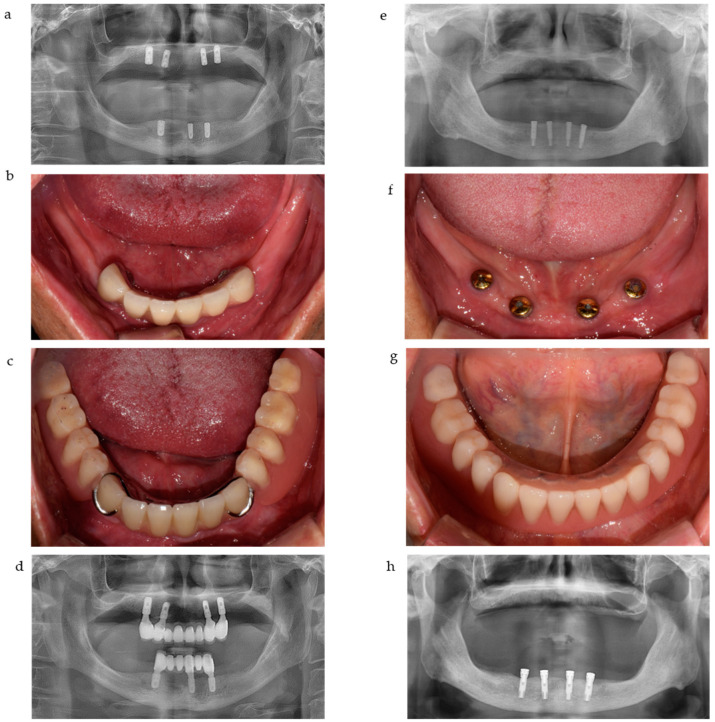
The representative cases of IC-RPD and IOD in this study. (a) Implants were placed in anterior positions due to anatomical limitations. (b) Intraoral view of IC-RPD case. (c) Delivery of IC-RPD. (d) Panoramic radiograph of IC-RPD case. (e) Four implants were placed in anterior positions due to anatomical limitations. (f) Intraoral view of IOD case. (g) Delivery of IOD. (h) Panoramic radiograph of IOD case.
